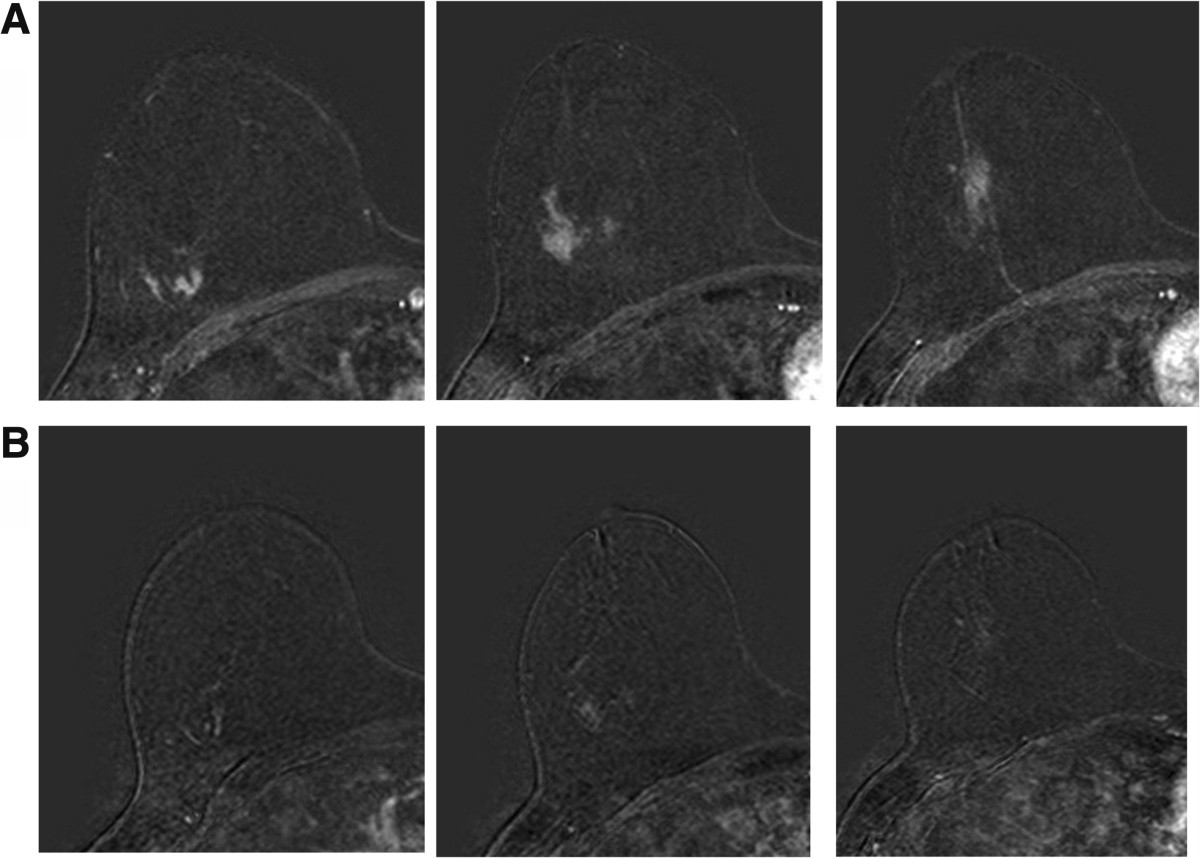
Treatment response assessed by MRI before (A) and after (B) lapatinib treatment.
